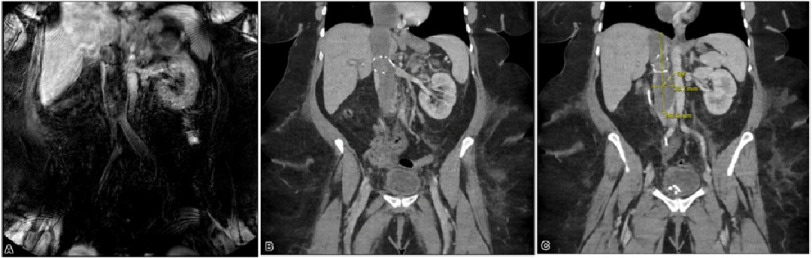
MRI venography showing occlusive bland inferior vena cava thrombus distending the IVC and extending to the right atrium and ventricle (A). CT scan depicting the dilated inferior vena cava, with IVC filter at the superior aspect. Findings are suspicious for a non-obstructing thrombus at this level (B,C).
